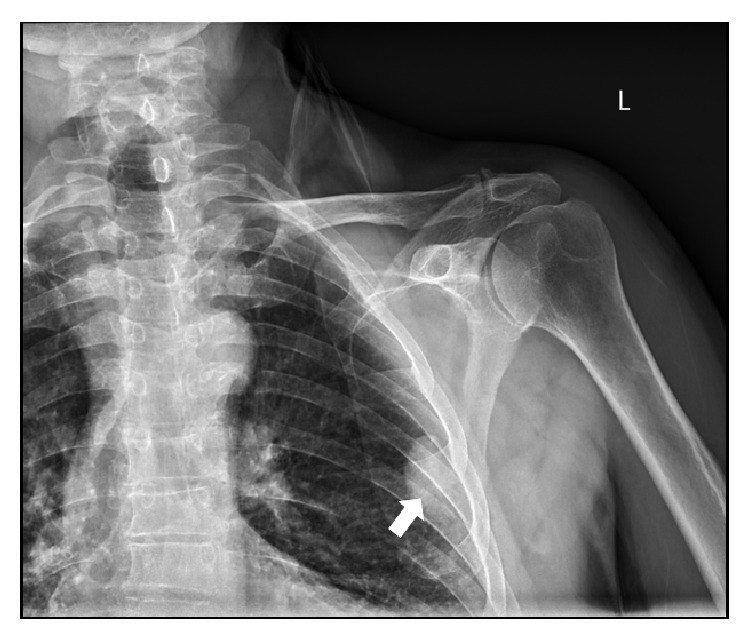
Anteroposterior shoulder X-ray showing left sided pleural-based mass.
